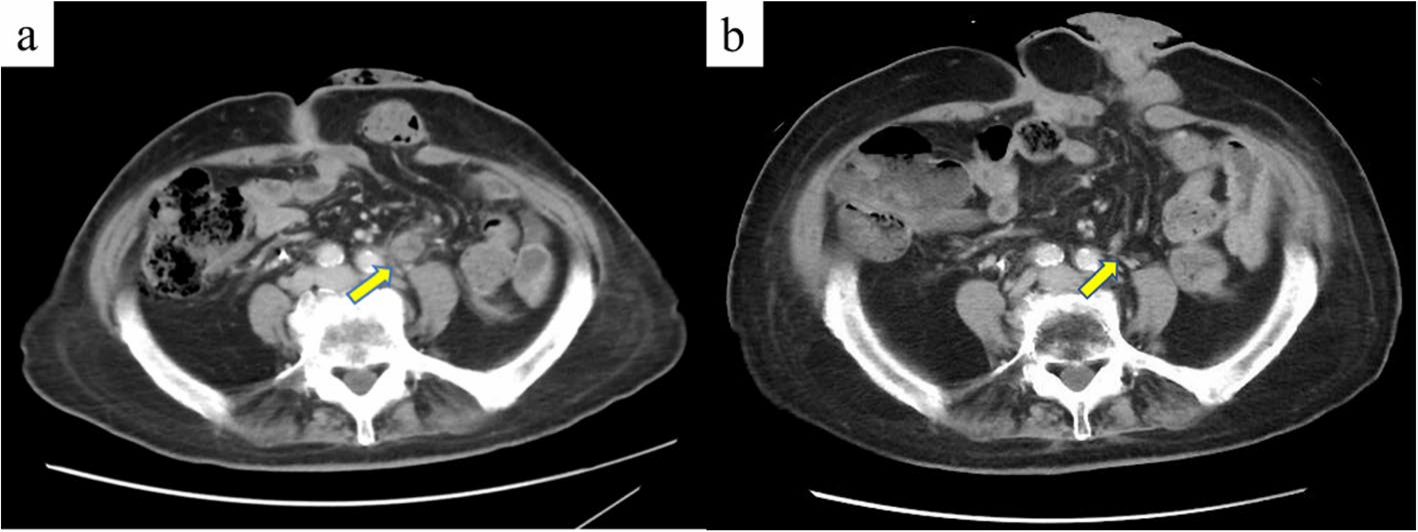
Contrast-enhanced computed tomography shows pelvic lymph node recurrence (a, indicated by an arrow). After radiotherapy, the metastatic lymph nodes disappeared (b, indicated by an arrow)
